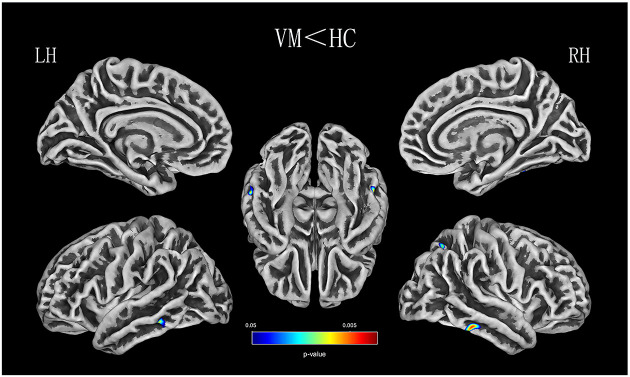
CT (thickness) analysis results of patients with vestibular migraine (VM) compared with healthy controls [*P* < 0.05, family-wise error (FWE)-corrected].
